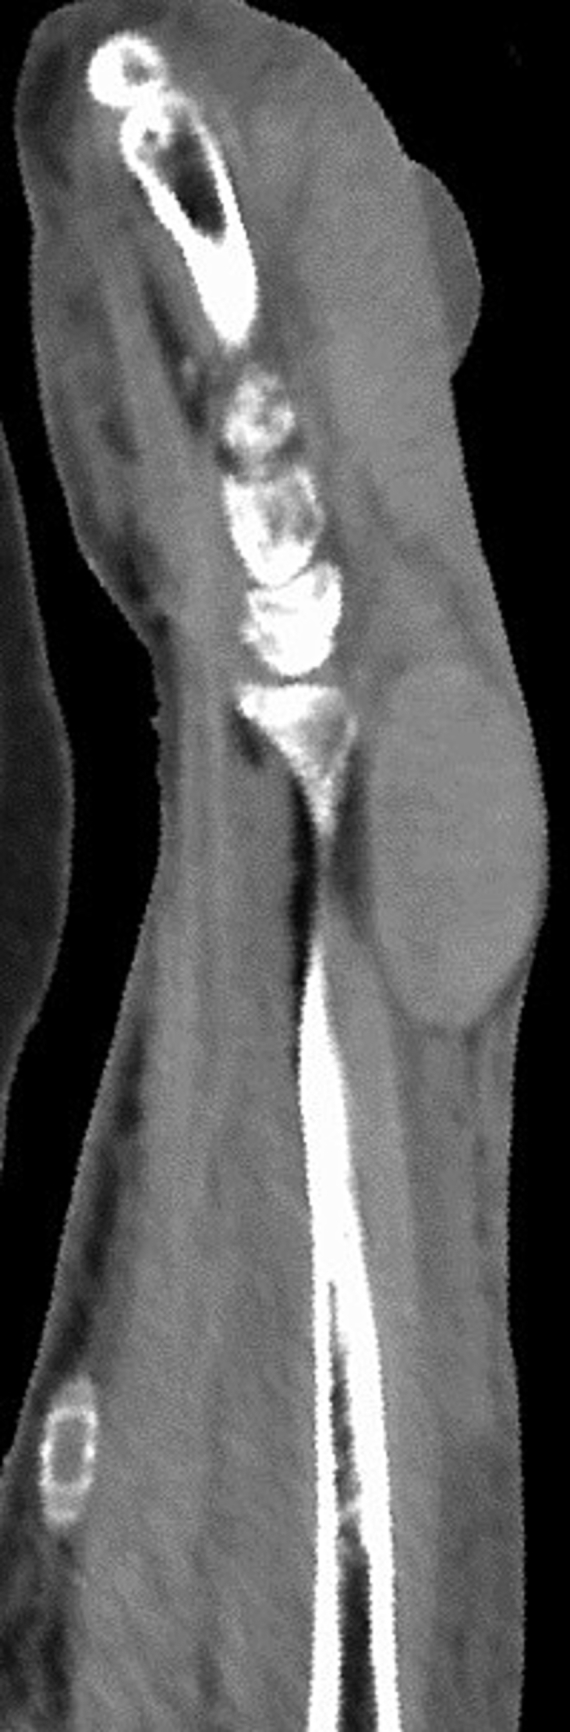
Computed tomography (CT) imaging shows a hematoma on the dorsal forearm.
